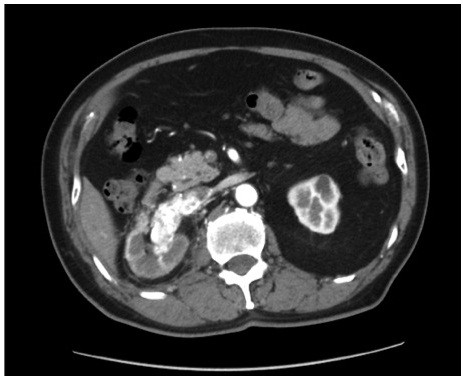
Enhanced computed tomography demonstrated an enhanced tumor thrombus in the inferior vena cava extending from the renal vein to the intrahepatic level.
